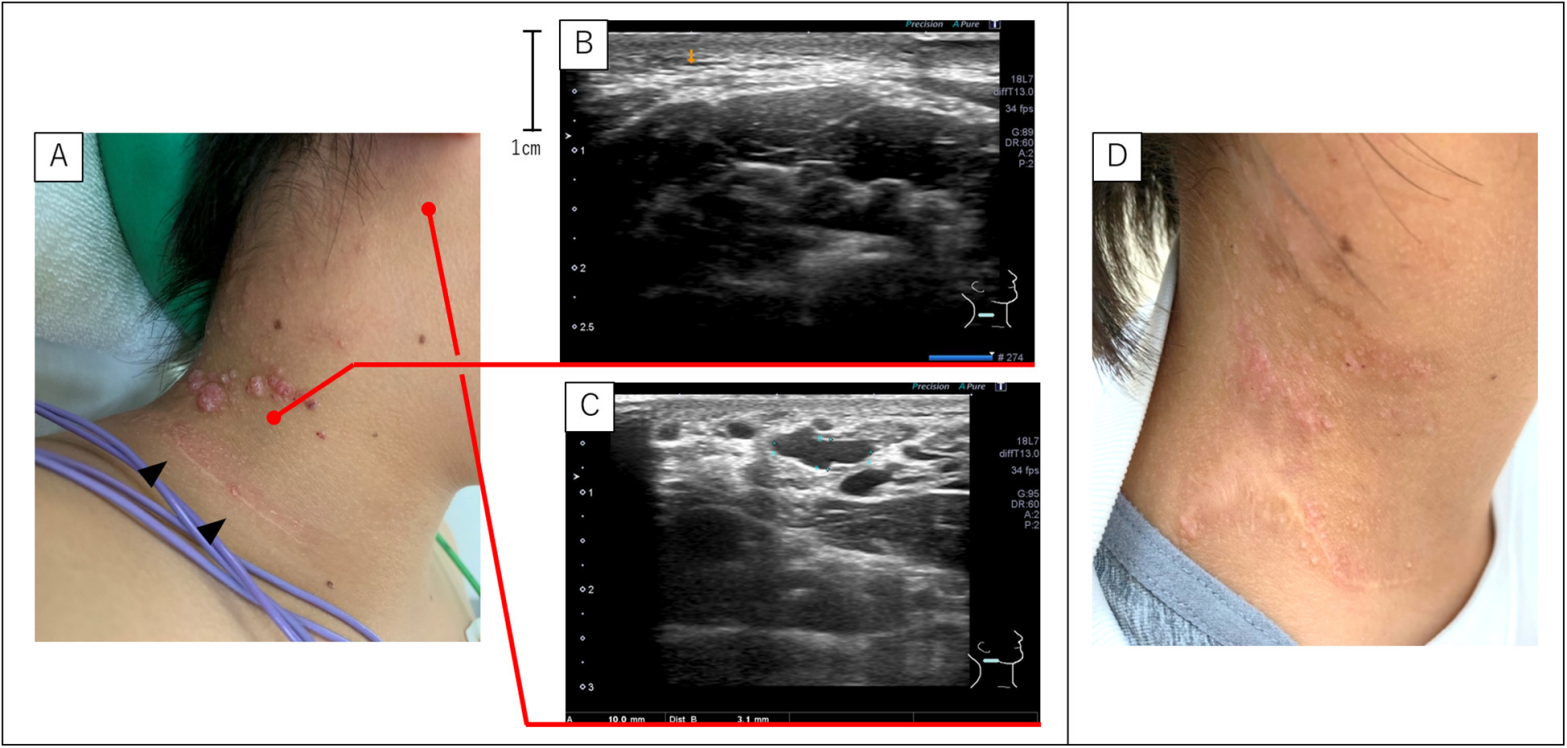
SLM and LM of case 3. (A) Gross appearance of the SLM before sclerotherapy. The SLM appeared on the cephalic side of the surgical scar (black arrowheads) at the previous hospital. (B,C) Ultrasonography detected no LM directly under the SLM. However, the LM was present near the lower edge of the ear and distant from the SLM. (D) Post-sclerotherapy findings show that the SLM is almost unidentifiable. LM, lymphatic malformations; SLM, superficial lymphatic malformation.
